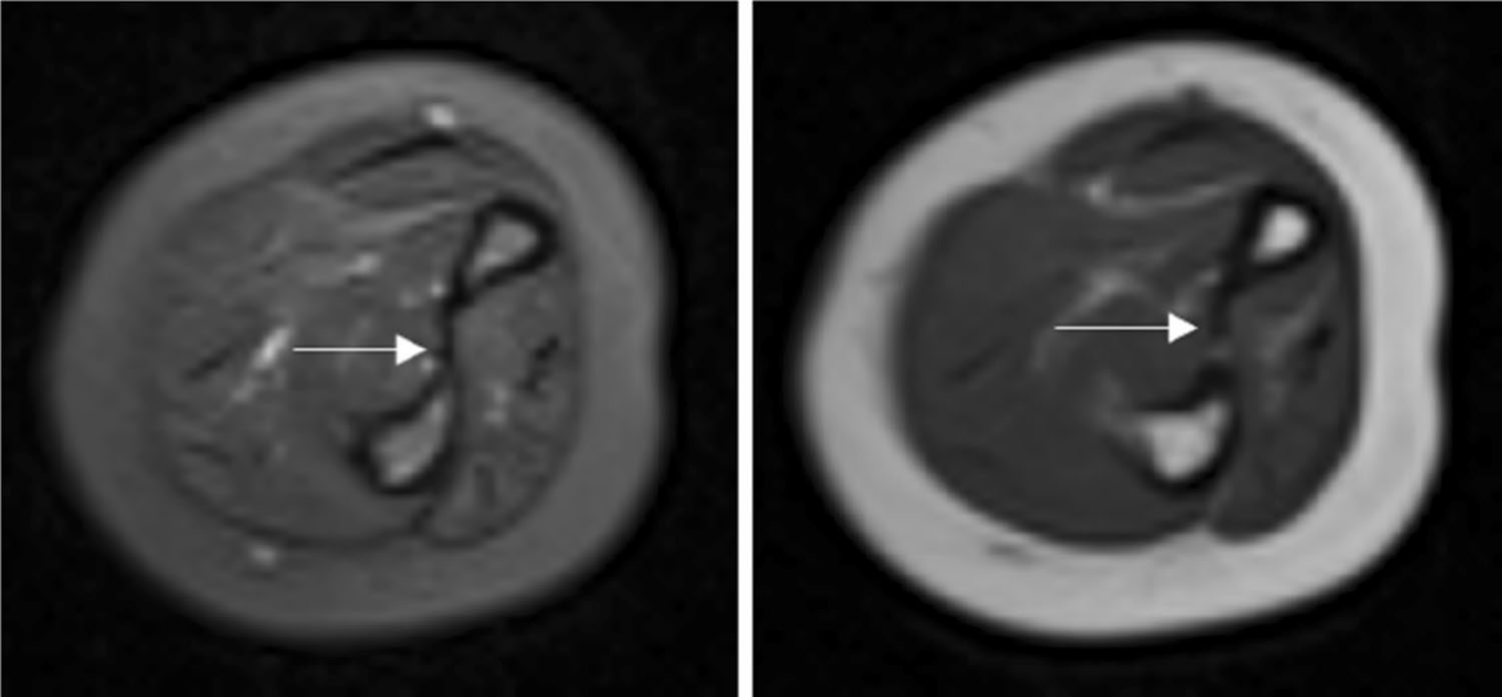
Axial T1 and T2-weighted images of the forearm (case 10) demonstrated no obvious the injuries of the interosseous membrane (the arrow).
